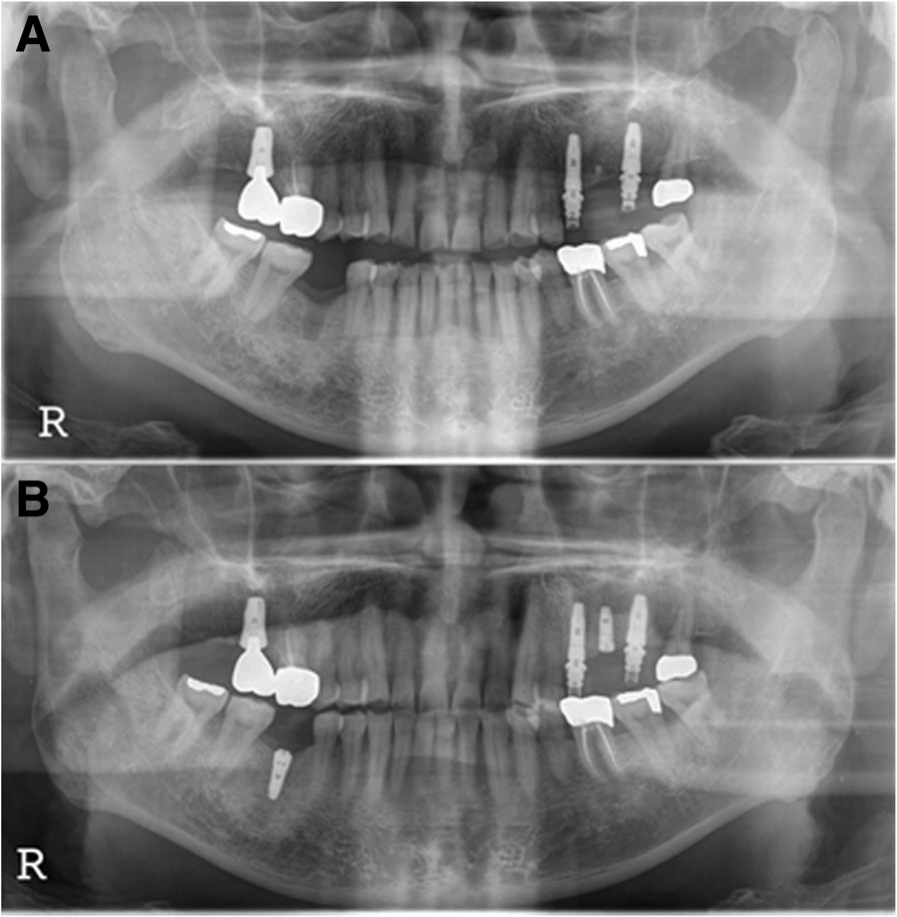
M3, panoramic radiograph, a postoperative 1-month removal of fixture state, b #46i, second installation
